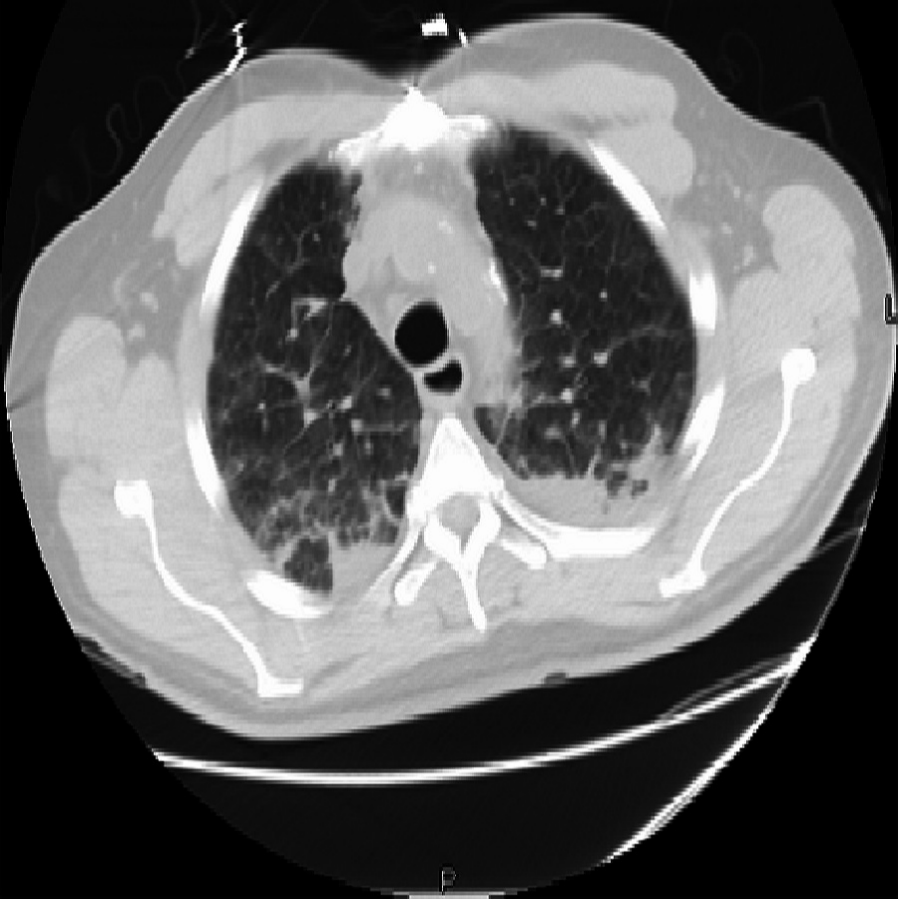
Computed tomography of the chest Ground glass changes in the mid-lung zones bilaterally, along with bilateral pleural effusions
